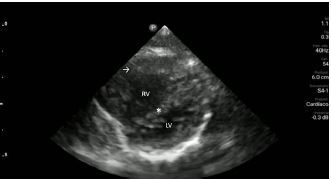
Parasternal short-axis view of the heart notable for interventricular septal flattening (*) due to right ventricular pressure overload in the setting of right ventricular hypertrophy (arrow). LV, left ventricle; RV, right ventricle.
